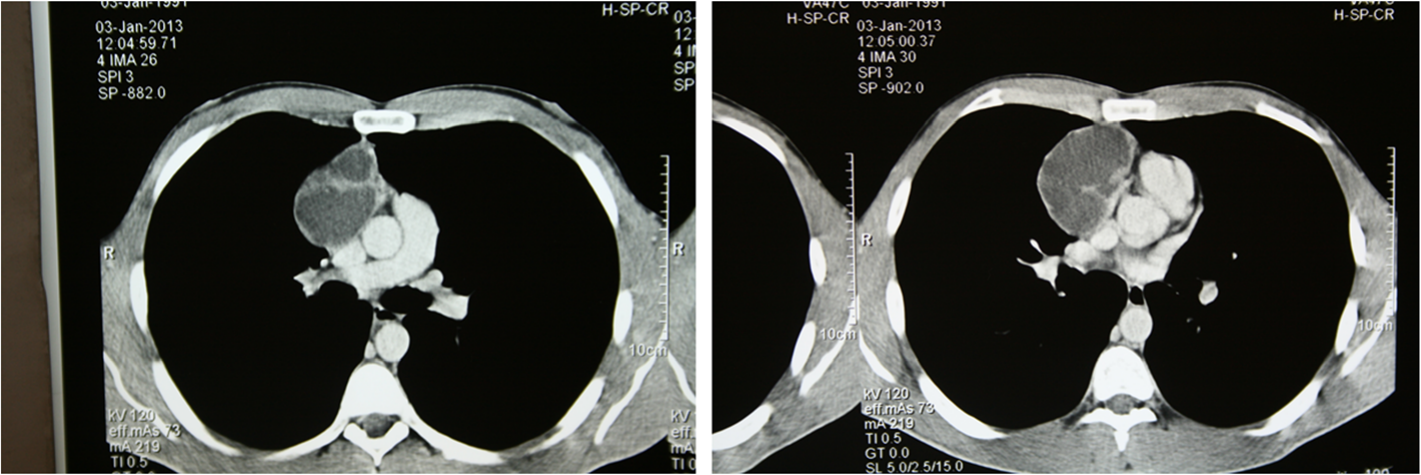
Chest CT (2013) shows an anterior mediastinal mass extending into the right side of the chest
